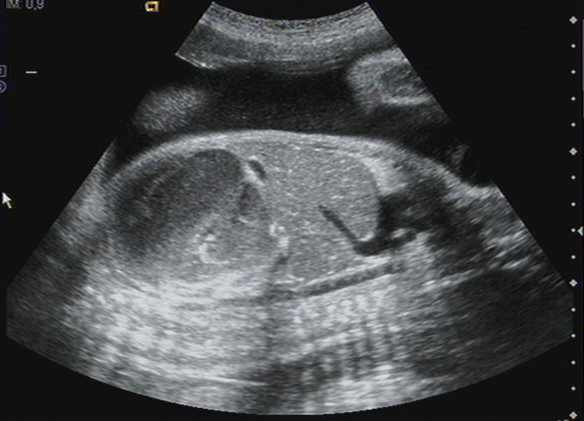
Ultrasound image: 32.3 weeks of gestation. Longitudinal scan image thorough the fetal abdomen identifying a mass occupying the entire left hemiabdomen (meconium pseudocyst), with mixed echogenicity. No calcifications were observed.
